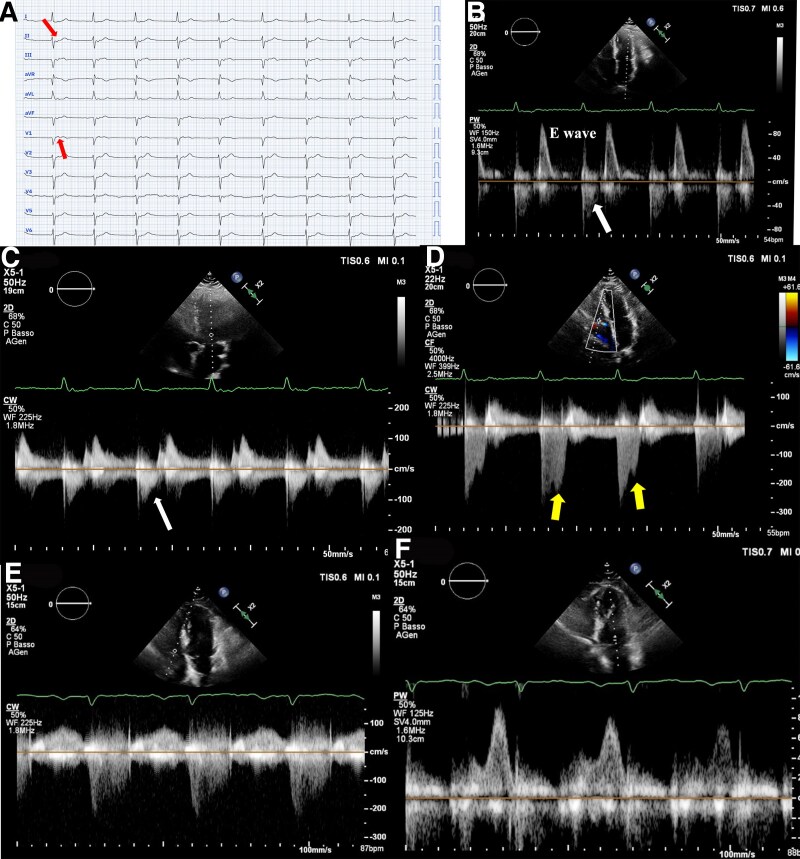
Mitral continuous wave (CW) Doppler (*Panel C*) shows a negative wave (white arrow) that synchronizes with the retrograde P-wave. *Panel D* presents tricuspid valve (TV) CW Doppler, where mild systolic tricuspid regurgitation (TR) is observed. Notably, a second regurgitant jet (arrows) occurs shortly after the retrograde atrial activation, in the later part of systole. This atrial retroactivation, due to the JR, prompts TV opening, and the pressure gradient between the right ventricle and right atrium causes the second jet. Interestingly, the TR jet resulting from atrial retroactivation is more pronounced than the mitral jet, likely due to the lower right ventricular pressure allowing a wider TV opening compared with the MV.
